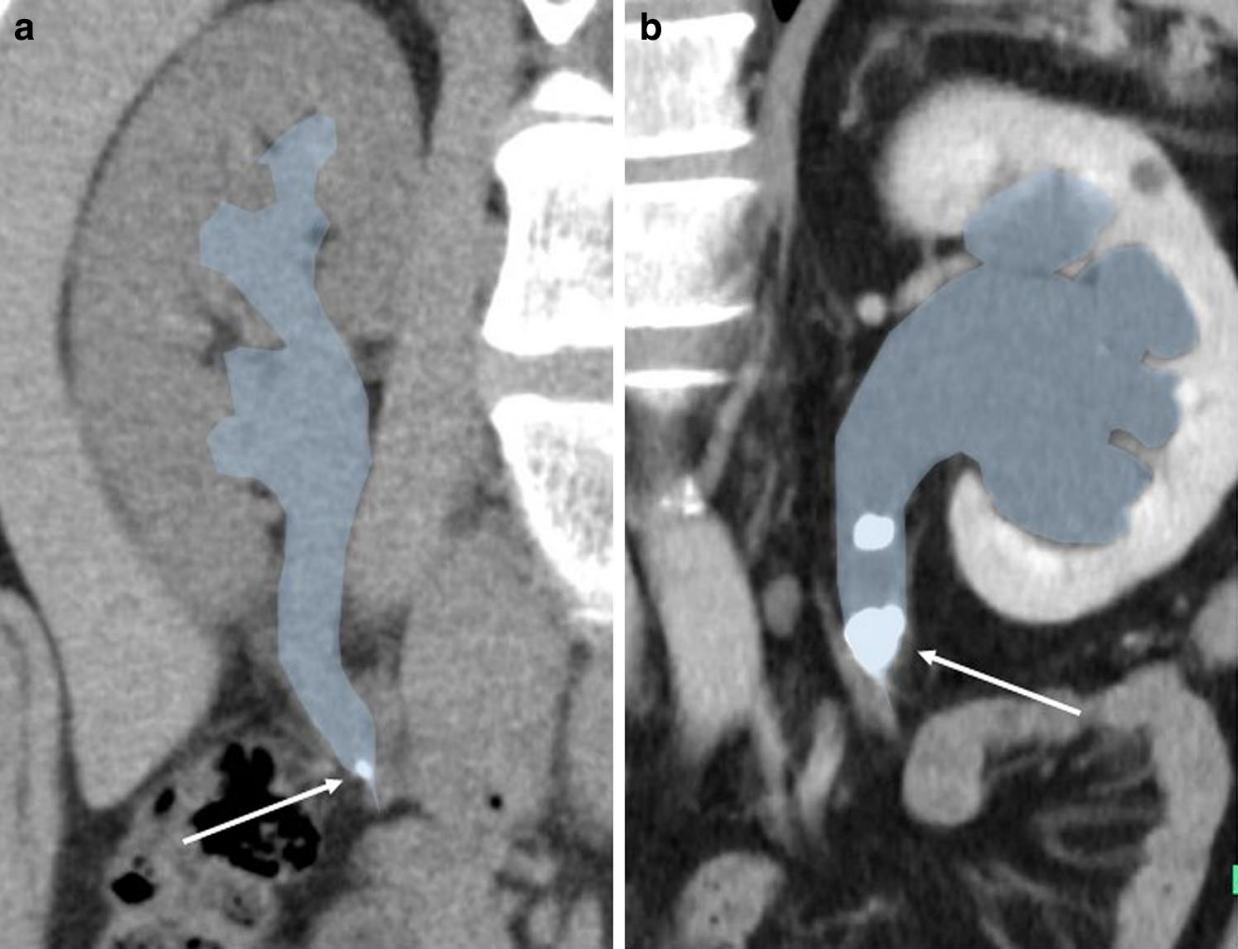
Oblique coronal computed tomography images of stones lodging at the level of the crossing point. a A 27-year-old male with a urinary stone at the level of the crossing point, b A 53-year-old male with a urinary stone at the level of the crossing point. When a stone lodges in the urinary tract (white arrows), the upstream tract dilates because of urinary stasis. Thereby, the crossing point mimics the ureteropelvic junction, the location where the diameter of the urinary tract originally changes
